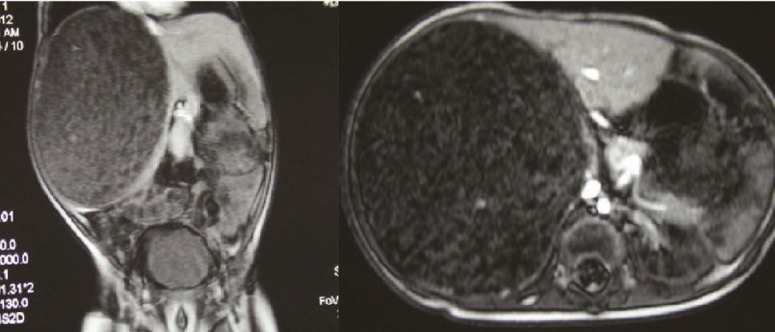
Abdomenopelvic MRI (sagittal and coronal) reveals a large well-defined heterogeneous mass measured 12×10 cm in the right lobe of liver causing displacement of and compression on the adjacent vessels and right kidney, in favor of hepatoblastoma
